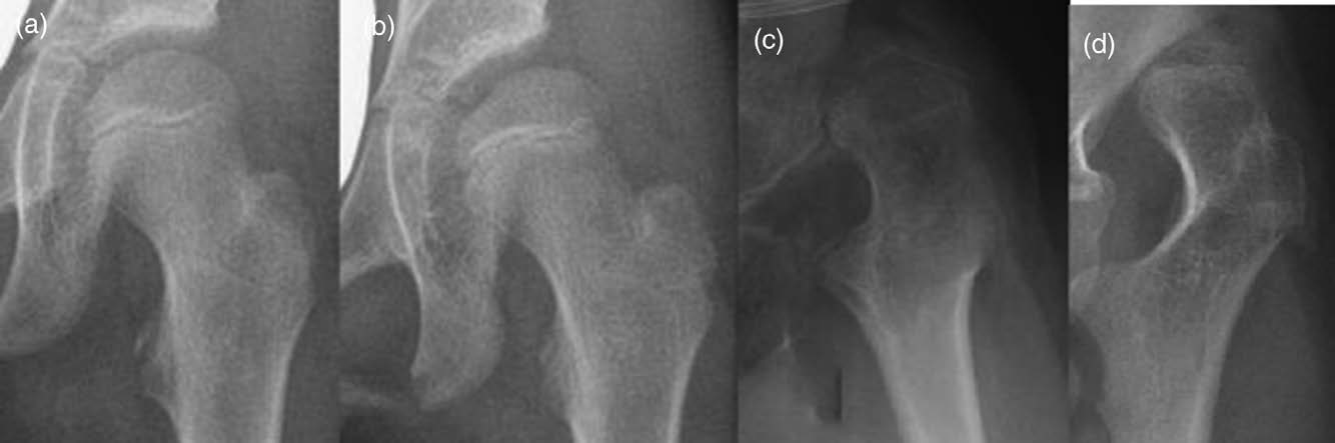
Femoral head shape classification described by Rutz *et al*. demonstrated on AP X-rays. AP, anteroposterior.
